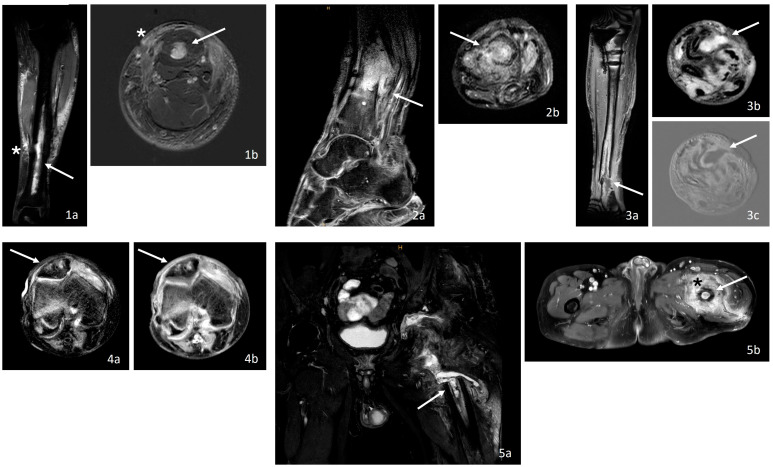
Typical osteomyelitis findings in MRI: bone marrow edema and intramedullary abscess (↑) with associated soft tissue reactions (*), also see Table 4. (1) MRI of the left lower leg with chronic osteomyelitis and bone marrow edema ((a): coronal, STIR TSE; (b): transverse, sT1w TSE). (2) MRI of the left ankle with chronic osteomyelitis including bone marrow edema and a developing intramedullary abscess ((a): sagittal, PDw mDIXON TSE; (b): transverse, T2w mDIXON TSE). (3) MRI of the left lower leg with acute-on-chronic osteomyelitis of the distal tibial shaft with intramedullary abscess ((a): coronal, T1w mDIXON TSE + contrast; (b): transverse, T2w mDIXON; (c): transverse, sT1w TSE). (4) MRI of the right knee with patella showing chronic osteomyelitis with intrapatellar edema and peripheral contrast enhancement as a sign of a developing intraosseous abscess ((a): transverse, T2w mDIXON; (b): transverse, T1w mDIXON TSE + contrast). (5) MRI of the left pelvis/hip with chronically abscessed osteomyelitis, bone marrow edema, and intramedullary abscess ((a): coronal, T2w TSE mDIXON; (b): transverse, T1w mDIXON TSE + contrast). mDIXON: modified DIXON, PDw: proton density-weighted, STIR: Short Tau Inversion Recovery, TSE: turbo spin echo, T1w: T1-weighted, T2w: T2-weighted.
